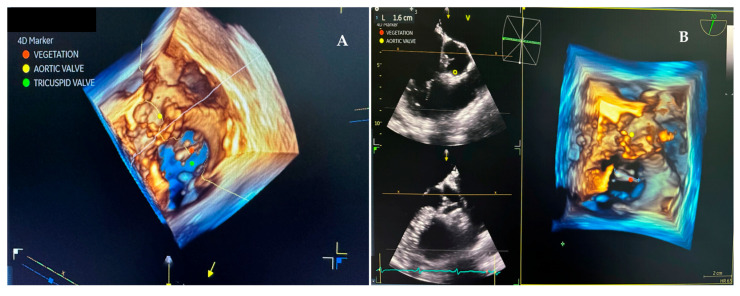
Transesophageal image (70°) RT3D acquisition. (A) Yellow dot—aortic valve; green dot—tricuspid valve; red dot—vegetation mass. (B) Yellow dot—aortic valve; red dot—vegetation measured 1.6 cm in length.
